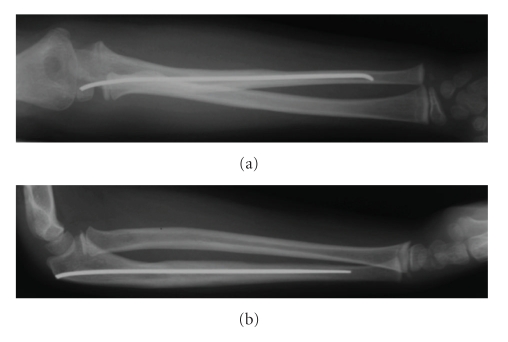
Anteroposterior (a) and lateral (b) radiographs of right forearm at six months showing fracture union and remodelling.
